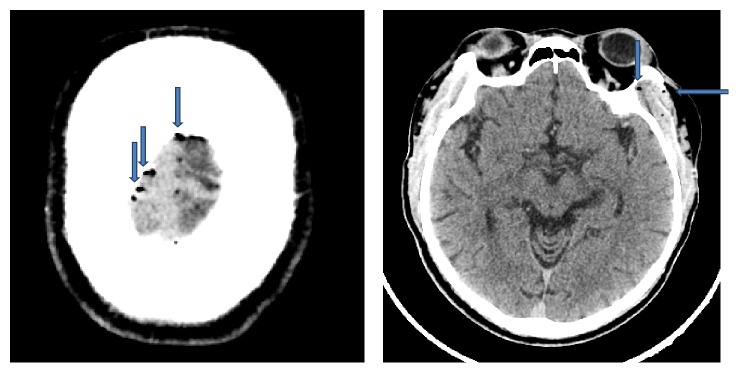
Case 1 CT scan of head: axial cuts showing foci of gas in cerebral vein and left temporal muscle (blue arrows).
